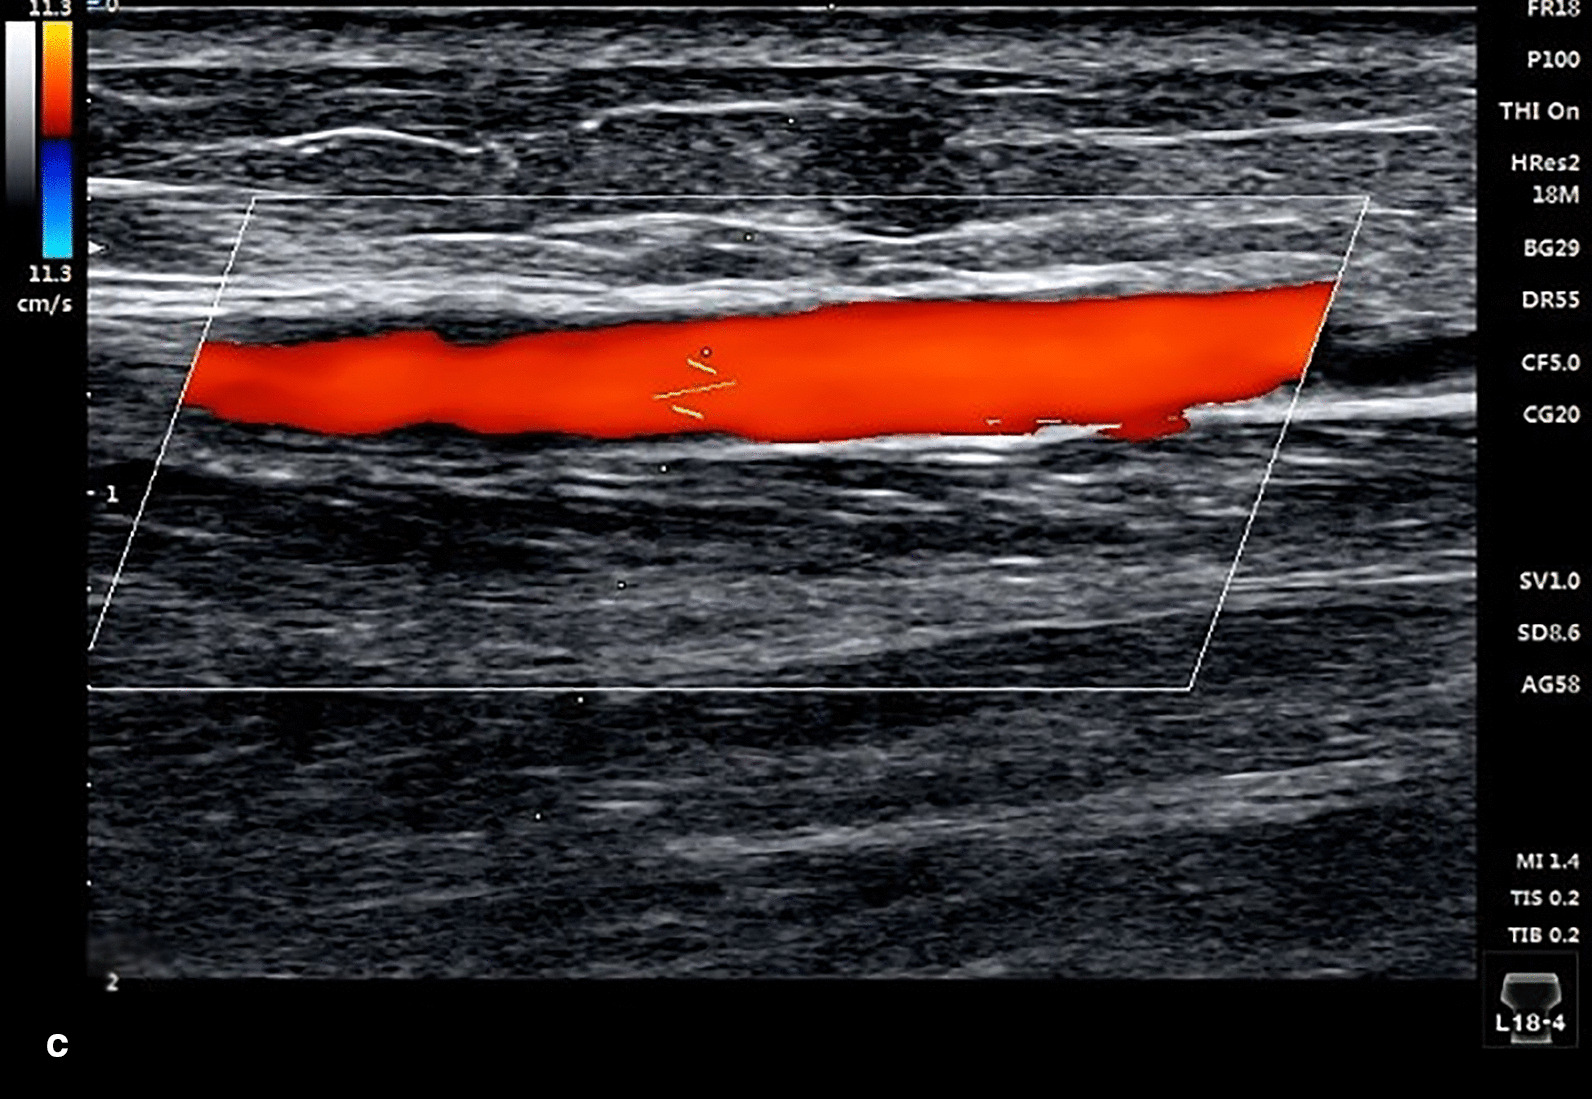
Ultrasonography of the blood flow resumed in the site of radial artery
